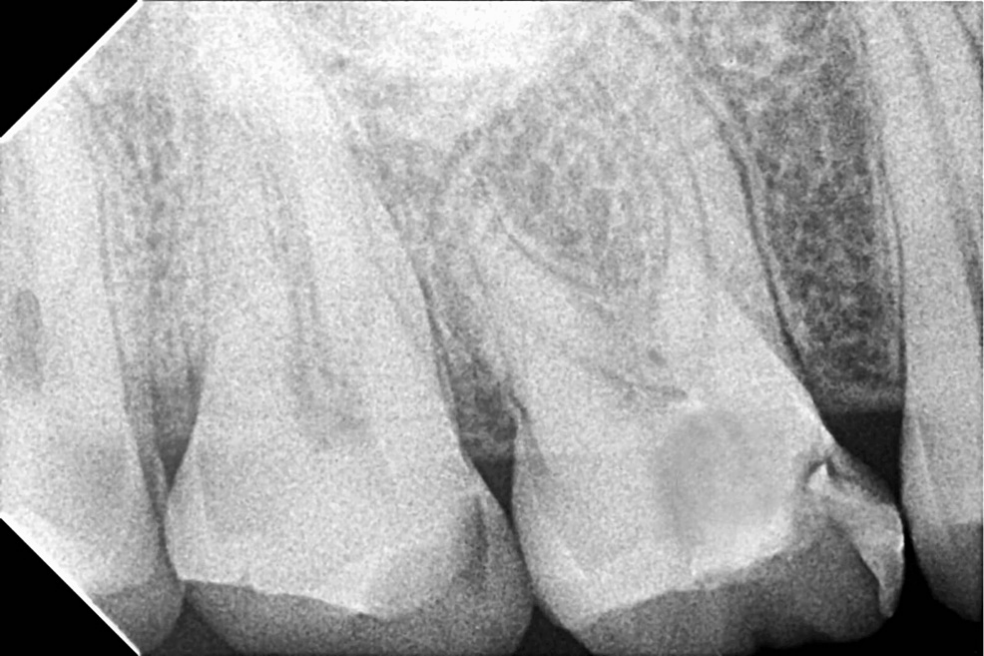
The figure shows a preoperative radiograph with the upper right first molar
